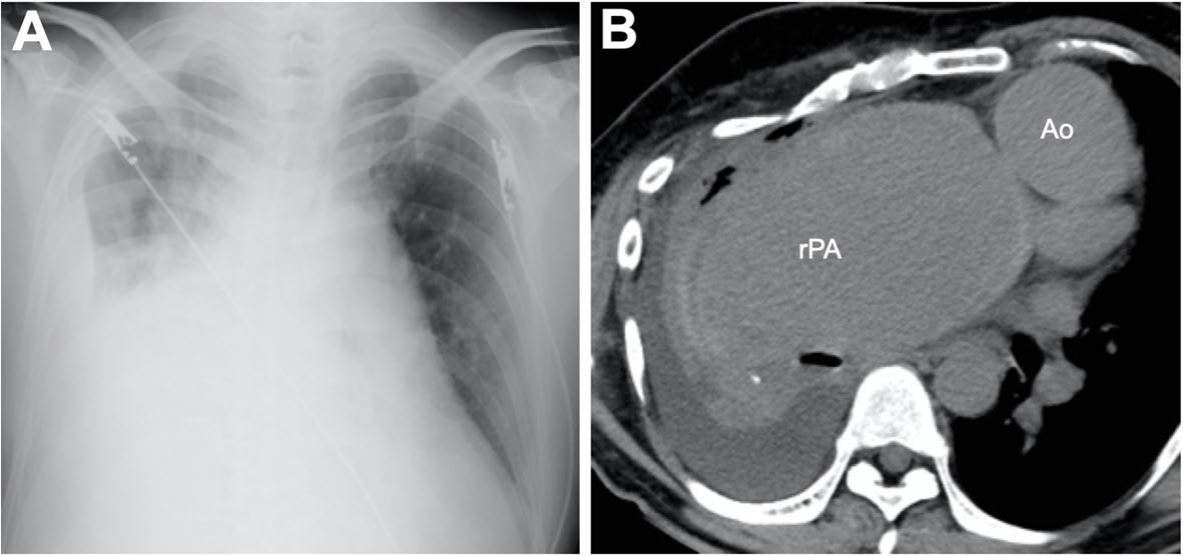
Impending rupture of pulmonary artery aneurysm. Large right pulmonary artery aneurysm and pulmonary effusion identified on A chest roentgenogram and B computed tomography. Ao, ascending aorta; rPA, right pulmonary artery
